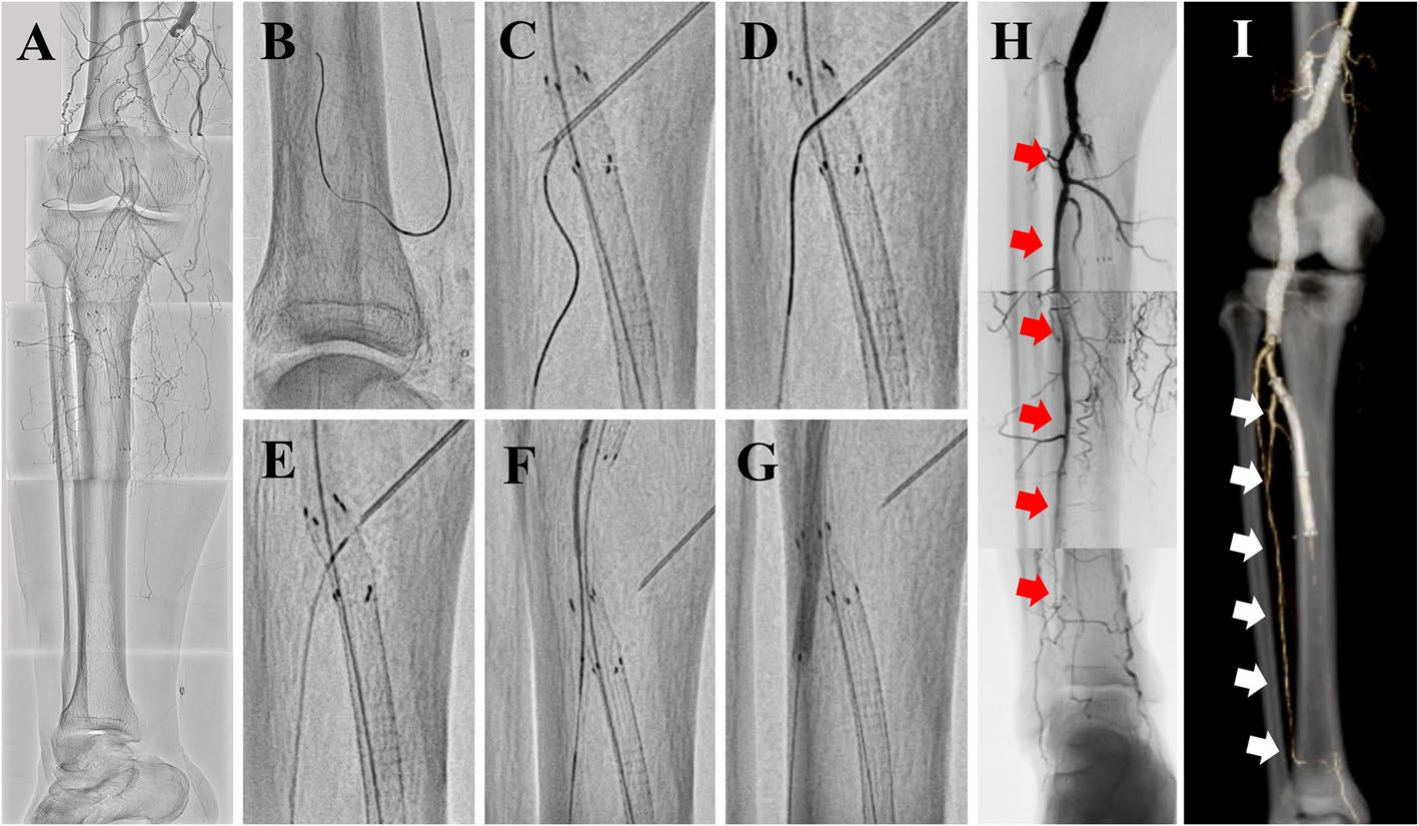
Overview and results of the ViaHole technique. A Initial angiogram showing thrombotic occlusion due to acute limb ischemia; B advancement of retrograde guidewire and microcatheter into the peroneal artery occlusion from distal posterior tibial artery via the posterior perforating branch of the peroneal artery; C successful puncture of the stent-graft with a 20-gauge needle, touching the tip of the retrograde microcatheter; D externalization of the retrograde guidewire through needle hole; E forceful advancement of the retrograde microcatheter over the externalized guidewire across the posterior wall of the stent-graft into the tibioperoneal trunk; F insertion of the antegrade guidewire into the retrograde microcatheter and advancement of the antegrade microcatheter into the peroneal artery; G dilation of the lesion, including the expanded polytetrafluoroethylene hole, using a 3.0 mm noncompliant balloon; H angiogram revealing successful creation of the stent-graft hole and sufficient flow from the peroneal artery (red arrows) to the below-the-ankle arteries; and I 1-year computed tomography angiogram demonstrating patency from the superficial femoral artery to the peroneal artery (white arrows)
